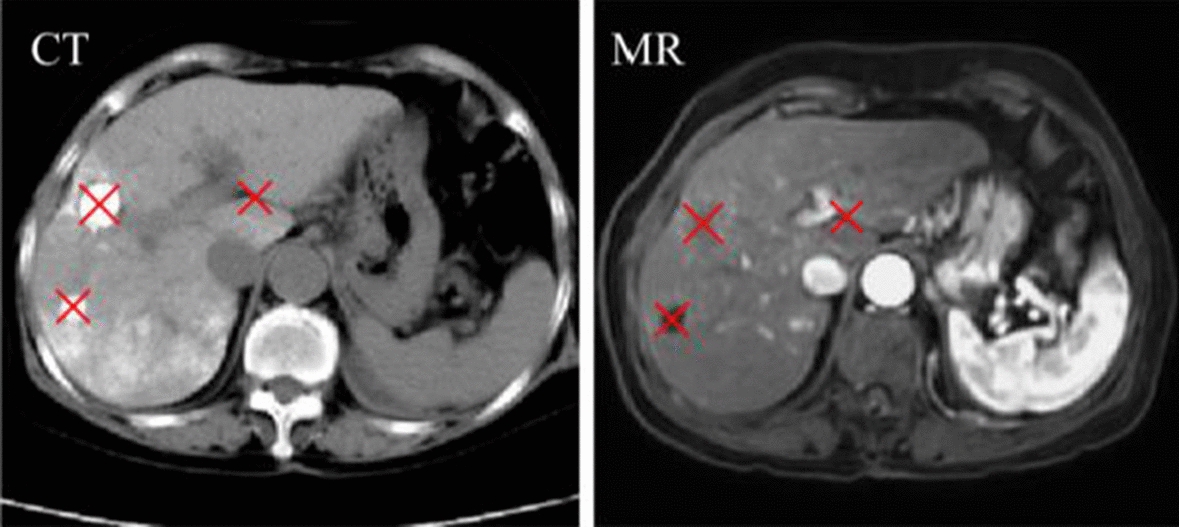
The identified landmarks (the central location of tumor and hepatic fissures) in CT and MR images. The TRE is defined as Euclidean distance between the corresponding landmarks
